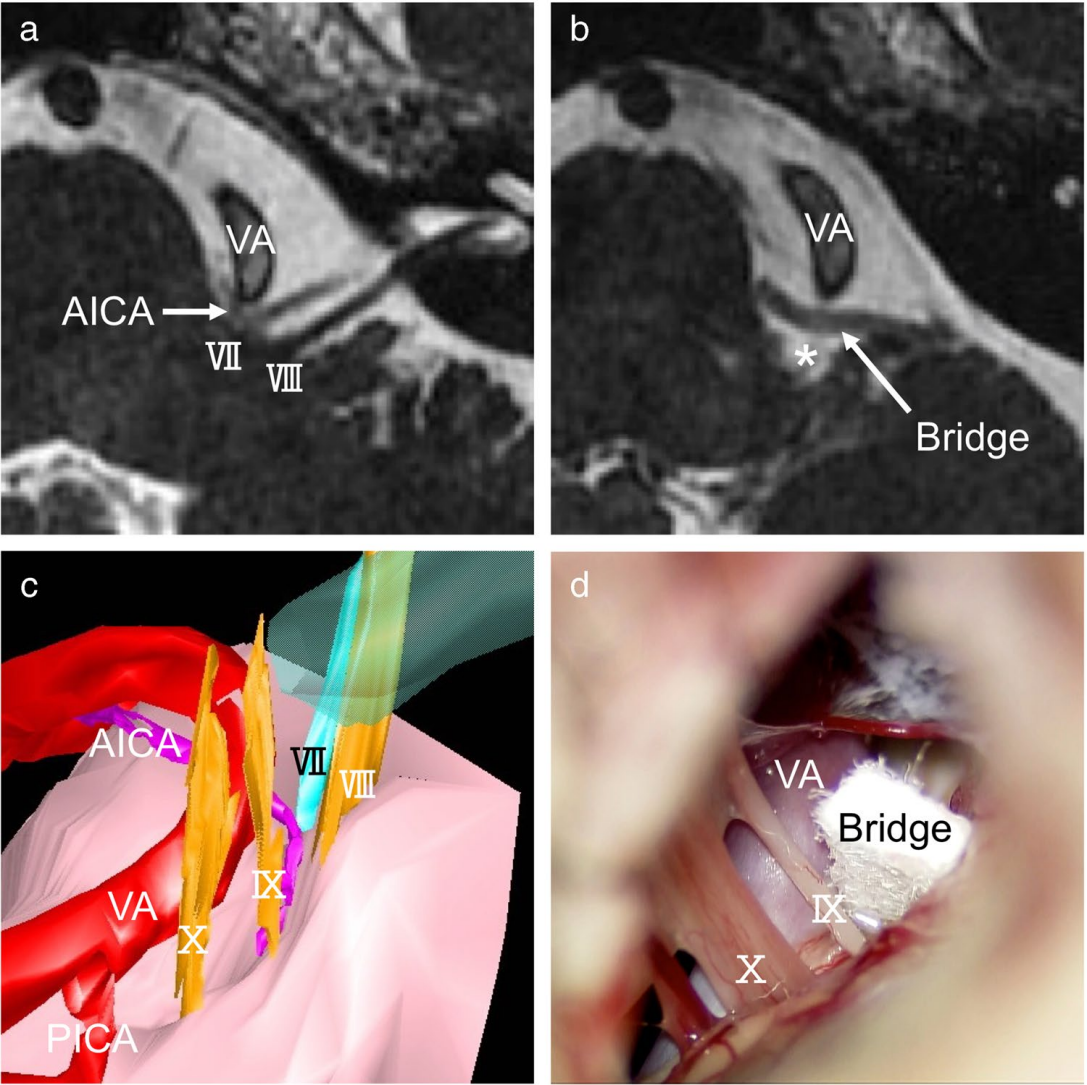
Case presentation (Case 2). A 54-year-old man with HFS on the left due to the VA and the AICA. a An MRI slice shows neurovascular compression by the AICA in combination with the VA on the facial nerve on the left side. b An MRI taken 1 year after MVD demonstrates the inserted bridge in place and a free space maintained over the REZ (asterisk). c A pre-operative three-dimensional (3D) image shows a relationship between the facial nerve and the adjacent structures. The AICA is the direct compression onto the REZ. The VA should be repositioned simultaneously to obtain sufficient decompression. d An intraoperative photograph indicating a single Teflon bridge inserted just above the ninth cranial nerve root to reposition the offenders, the VA, and the AICA. The lateral edge is placed on the flocculus, and the other edge is placed on the brainstem near the exit of the abducens nerve
